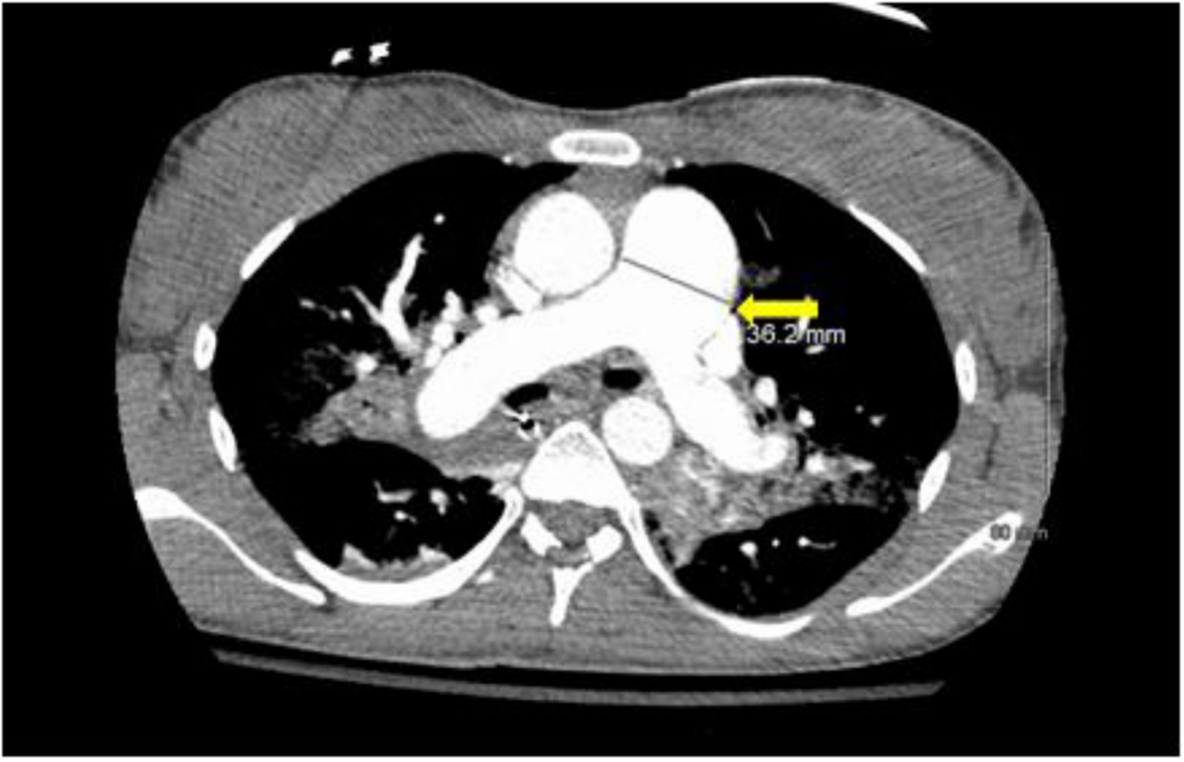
CT scan of the chest with contrast. The yellow arrow depicts enlarged main pulmonary arterial trunk
